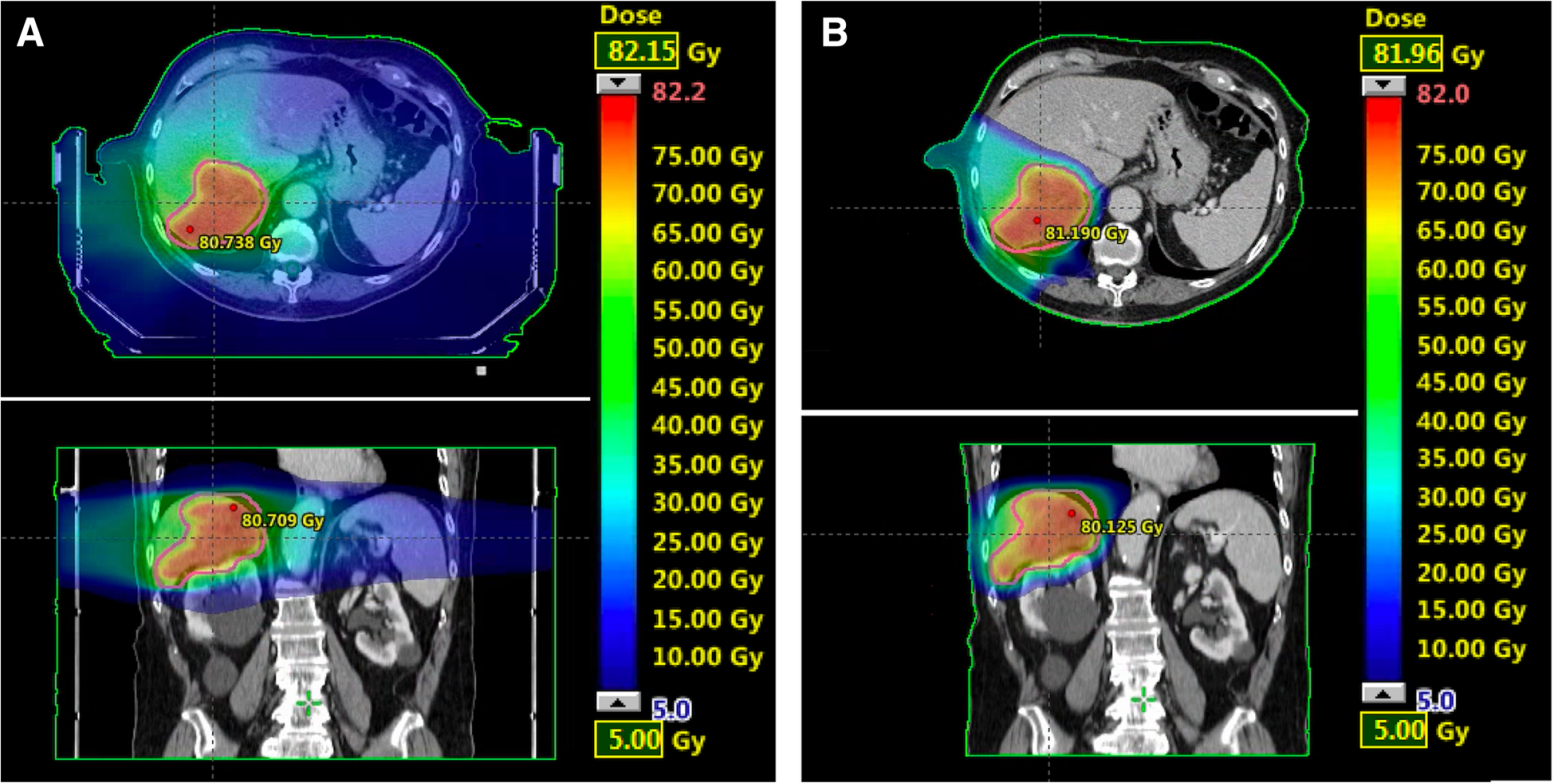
Dose distributions on axial and coronal CT-slices for patient #3 for (a) VMAT and (b) IMPT
